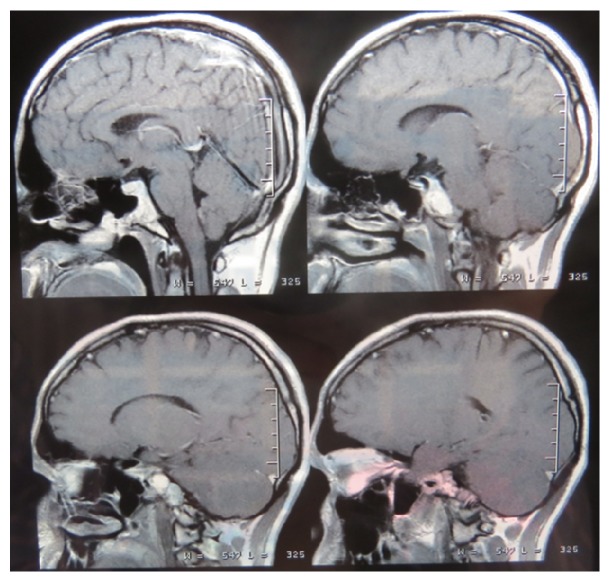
T1 weighted magnetic resonance imaging scans show resolution of the lesion.
